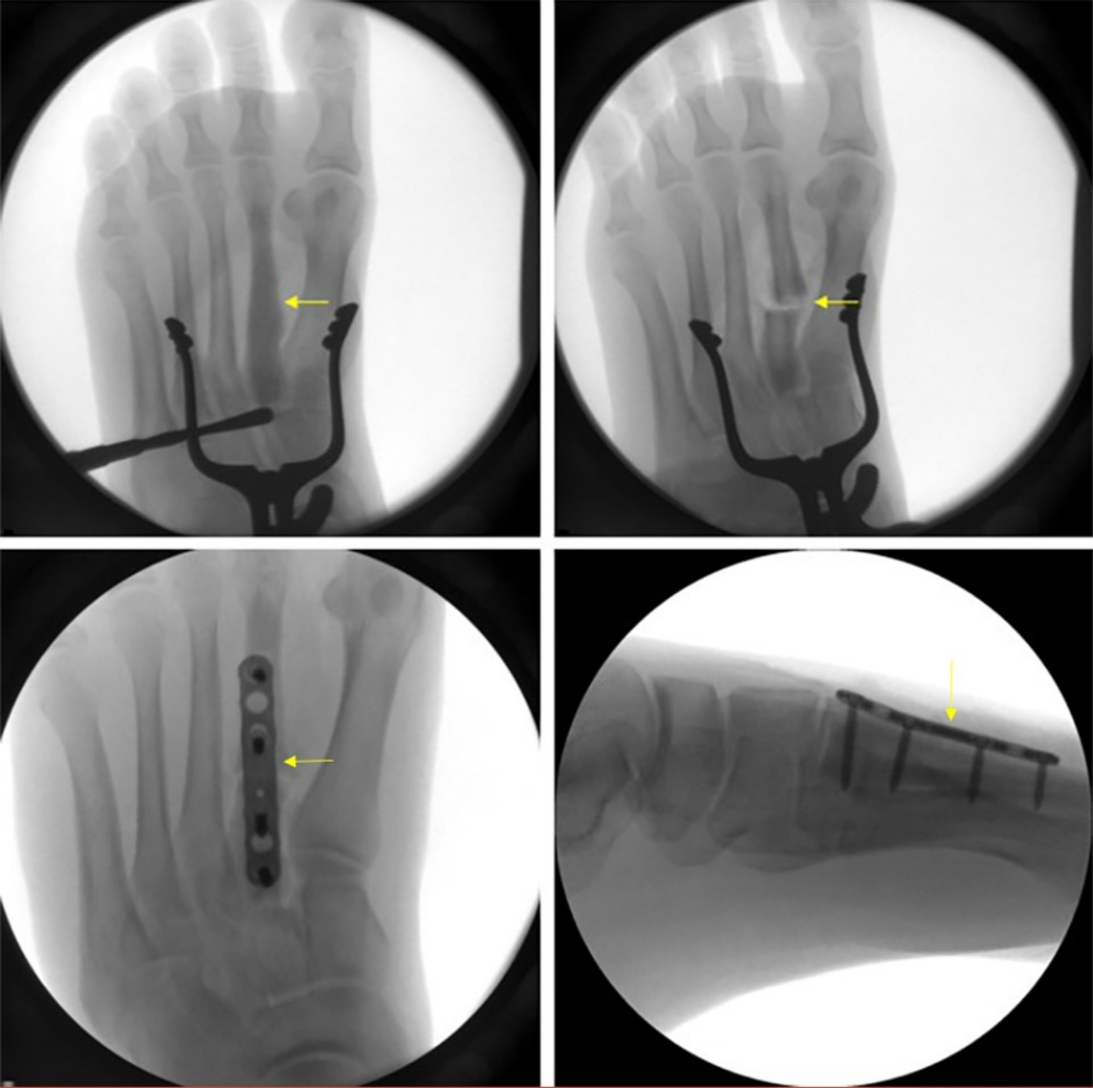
Intraoperative X-rays. Top row, left to right: cement in second metatarsal prior to open reduction and internal fixation; osteotomized second metatarsal in preparation for curettage and fixation. Bottom row, left to right: coronal view of metatarsal fixed with plate and screws; sagittal view of metatarsal fixed with plate and screws.
